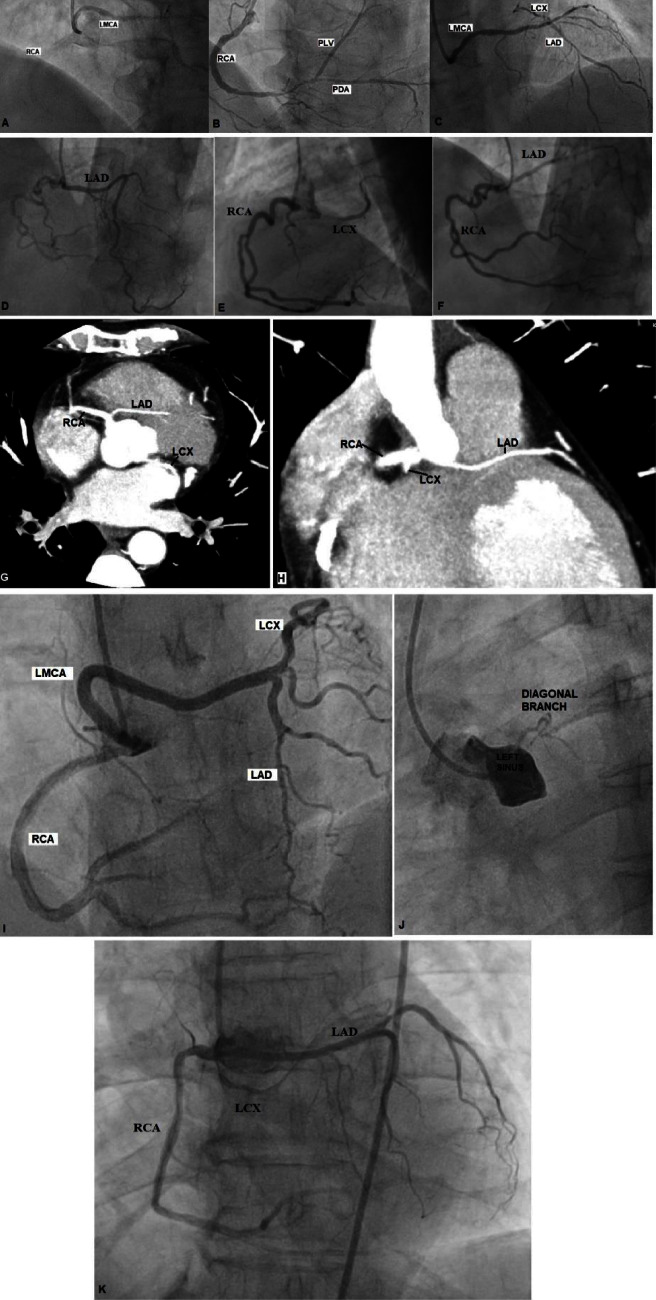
(a)–(c): CAG showing the origin of all 3 major coronaries from the right sinus of Valsalva. Dominant RCA showing 80–90% long tubular stenosis in mid to distal part along with 80% stenosis in proximal PDA. LMCA originates from the right sinus via common ostium with RCA and coursed retroaortic and then divided into LAD and LCX. LAD had long segment calcific 80% stenosis and LCX was distal diseased. (Case 3). (d)–(f): CAG showing single vessel disease with all 3 major coronaries originating from the right sinus via common ostium. LCX ran retroaortic with ostioproximal 95% thrombotic occlusion. LAD had mild plaque. Dominant RCA had 60% stenosis in the mid part. (Case 4). (g) & (h): CT coronary showing all 3 major coronaries LAD, LCX, and RCA arising from the right sinus via the common trunk. RCA showed 90% stenosis in the mid part. (Case 5). (i) & (j): CAG showing anomalous origin of LMCA from the right sinus along with RCA via common ostium. The left main coronary traversed retroaortic towards the left side and divided into the LAD and LCX branches. A small diagonal branch originated from the left sinus. (Case 6). (k): CAG showing all 3 major coronaries LAD, LCX, and RCA originating from the right coronary cusp via the common ostium. (Case 7).
